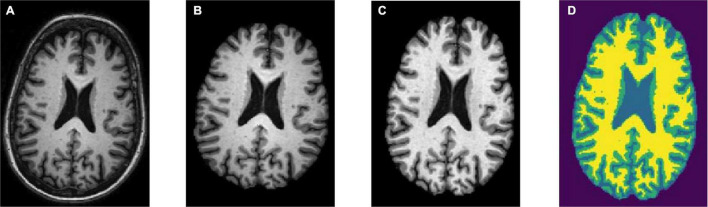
Preprocessing steps: (A) raw bran MRI, (B) skull stripped MRI, (C) bias field corrected MRI, and (D) tissue segmented MRI (WM is denoted in yellow, GM is denoted in green, and CSF is denoted in light blue).
